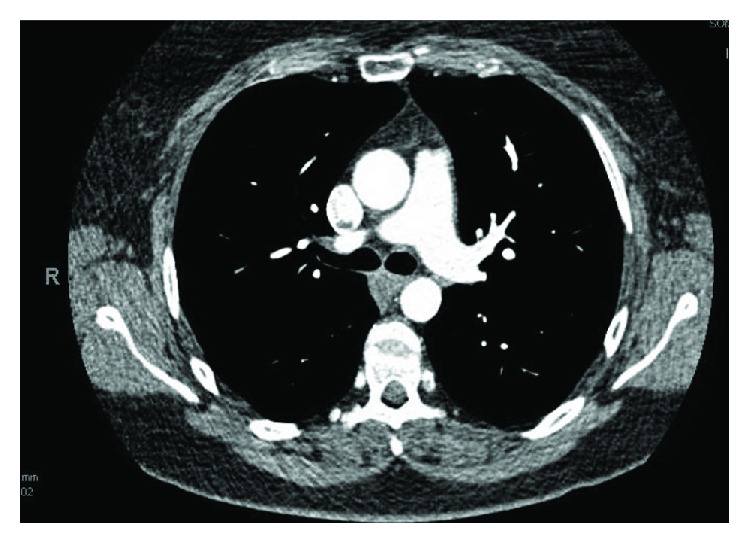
CT obtained for follow-up which reveals resolution of previously noted thrombus of the thoracic aorta.
